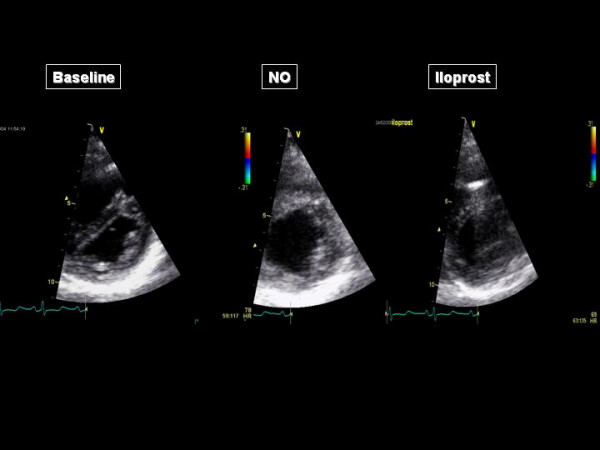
Parasternal short axis view before (left), during nitric oxide (NO) inhalation (middle) and during iloprost inhalation (right).
